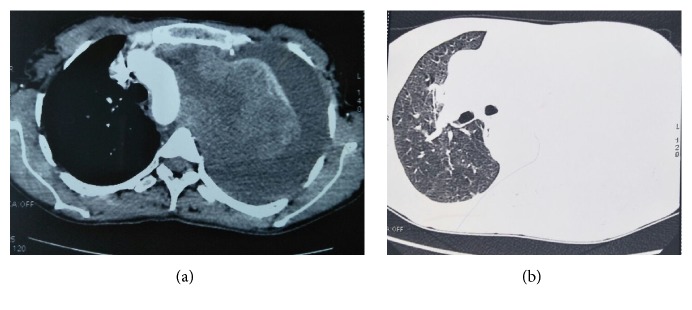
CT scan image showing a left mediastinal-pulmonary tumor process [(a) mediastinal window. (b) Parenchymal window].
